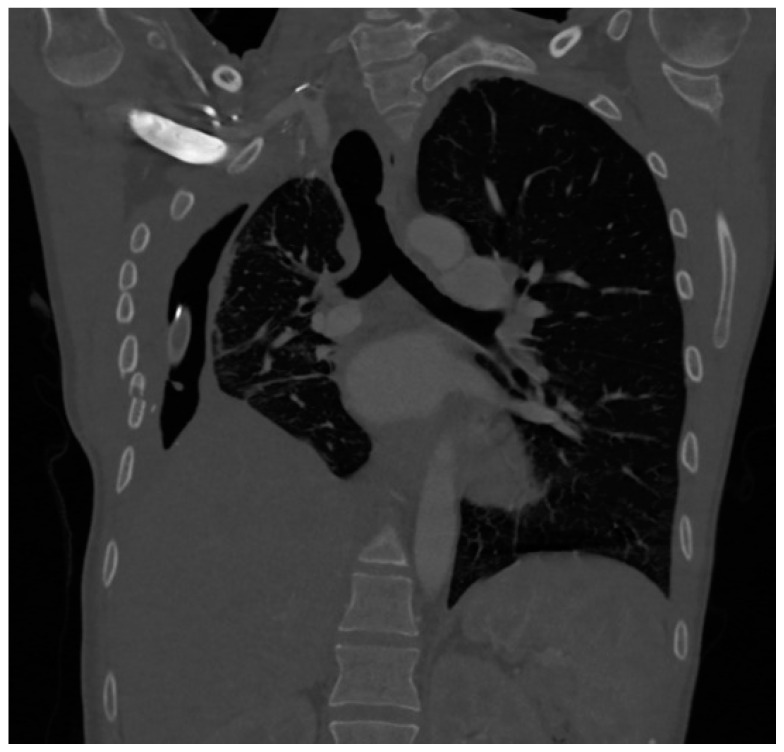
CT scan of the patient 2 at admission to the ICU.
